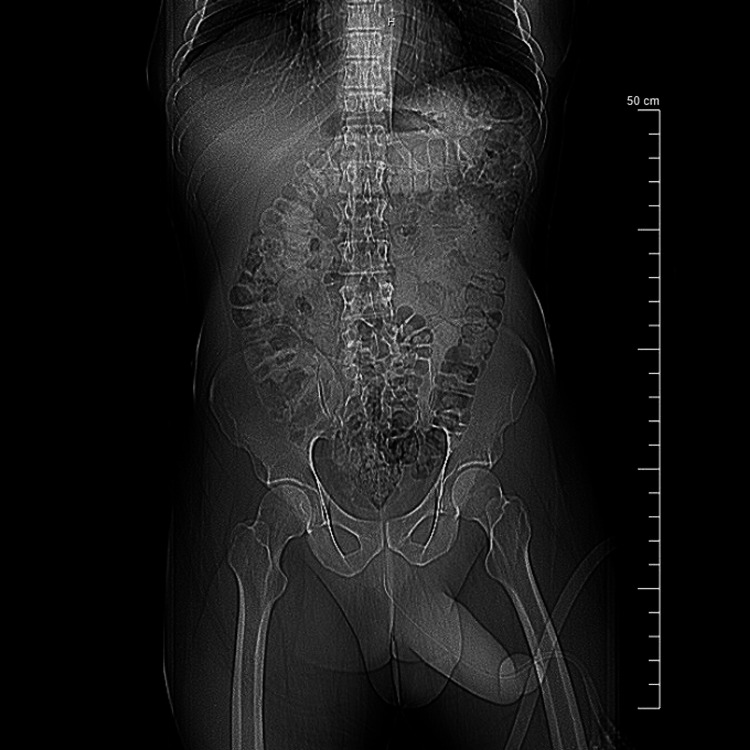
The CT scan of the abdomen and pelvis with contrast in the coronal view is unremarkable for malignancy or intra-abdominal masses. No large fluid collections are observed to suggest an abscess.
